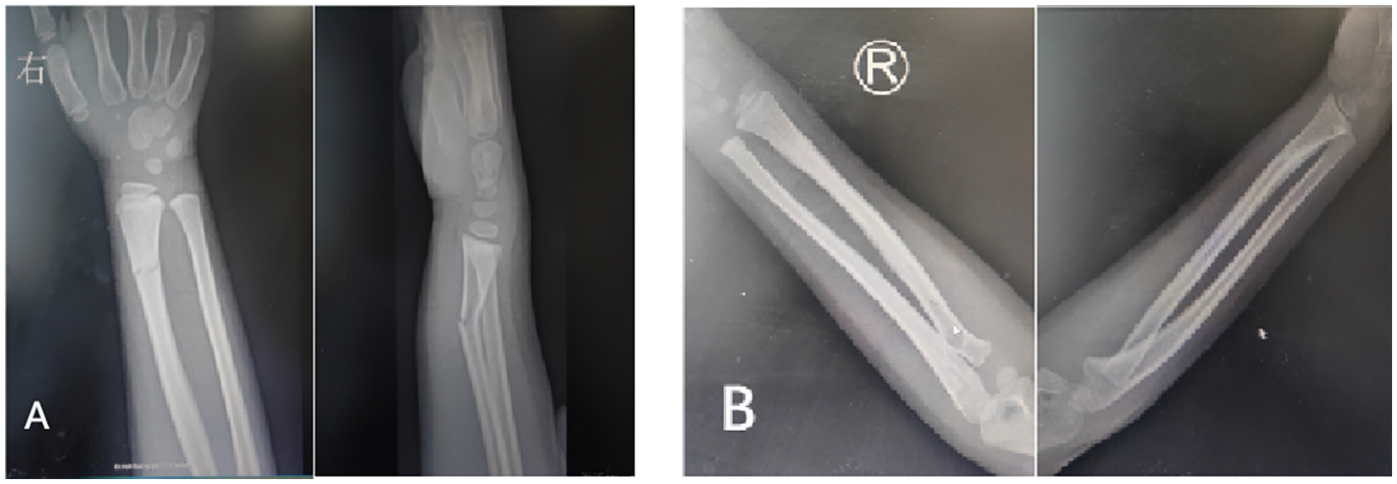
(A) X-ray after patient injury. (B) X-ray when patient uses 3D-printed splint for 4 weeks.
